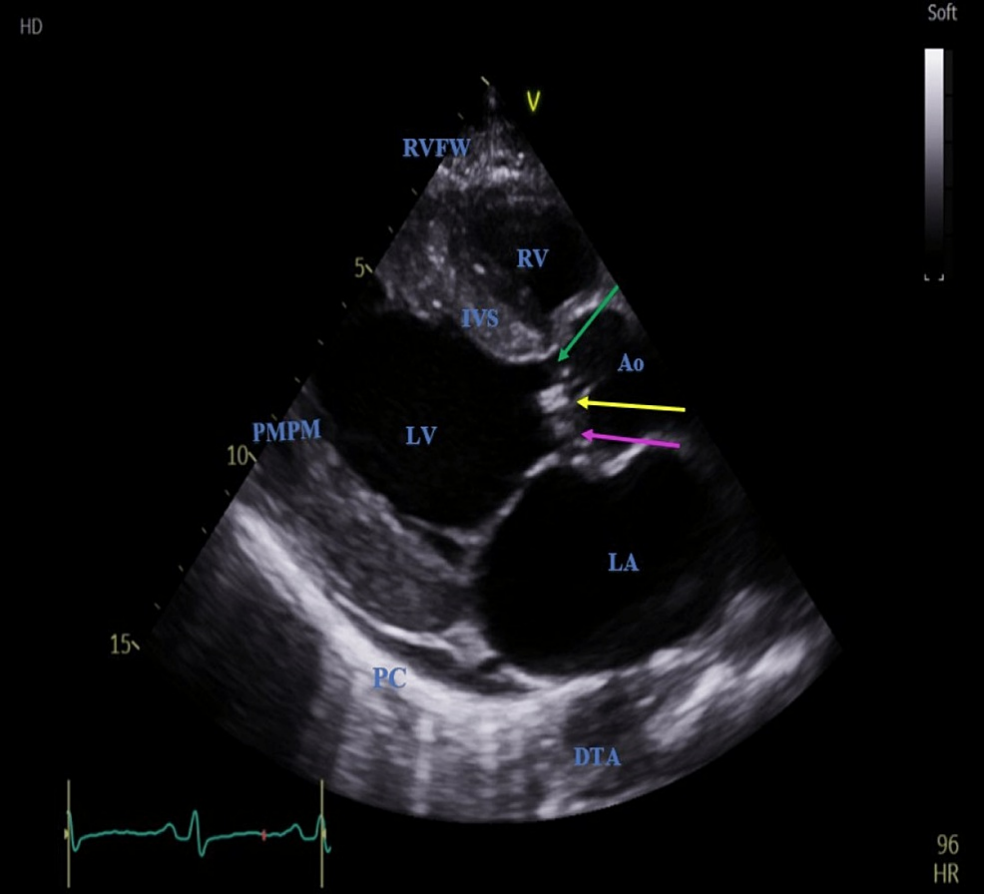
Parasternal long-axis view showing mobile, echogenic attachment on the aortic valve (yellow arrow) in between the right coronary cusp (green arrow) and non-coronary cusp (pink arrow) RV: right ventricle, RVFW: right ventricular free wall, IVS: interventricular septum, LV: left ventricle, PMPM: posteromedial papillary muscle, Ao: ascending aorta, LA: left atrium, PC: pericardium, DTA: descending thoracic aorta
